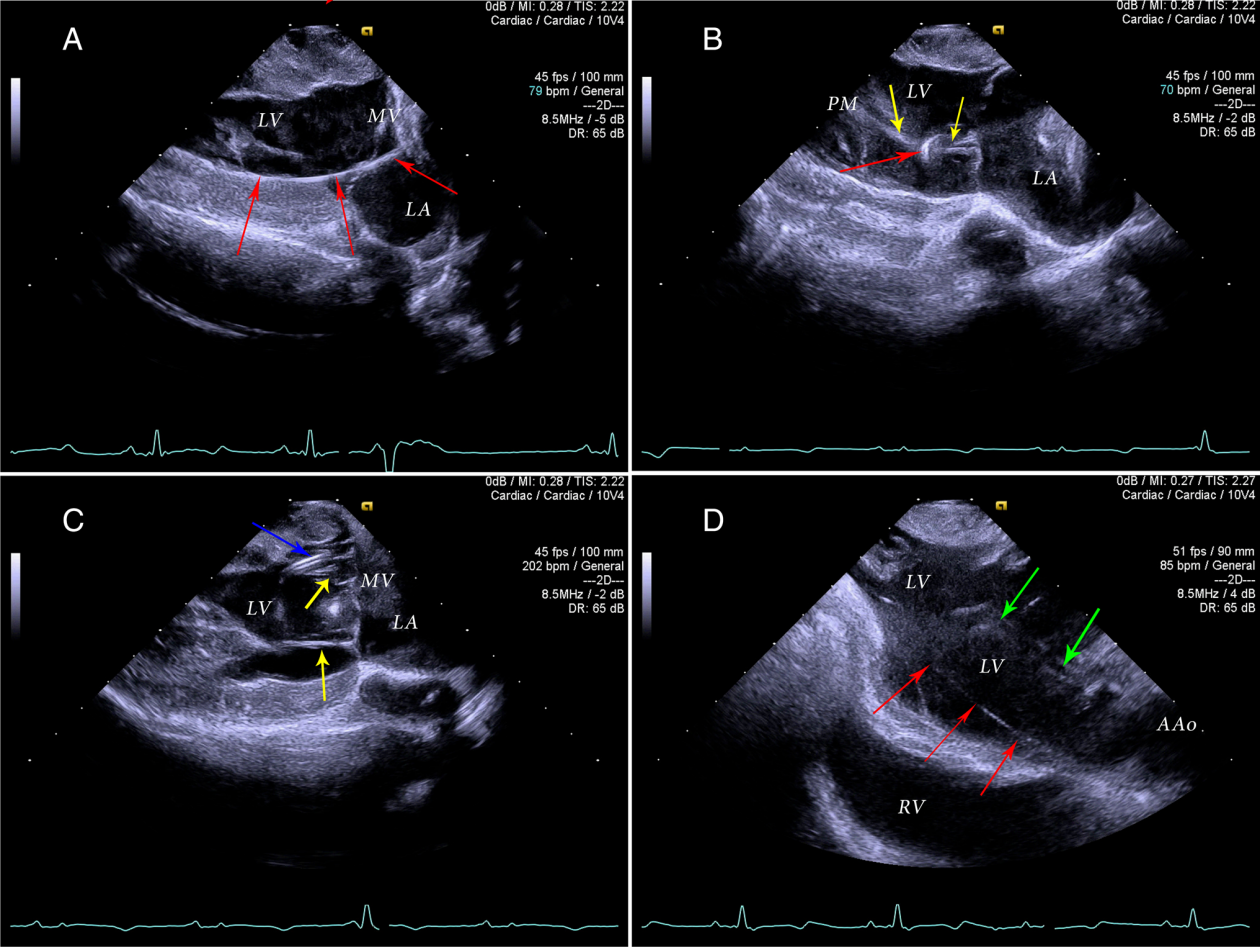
Echocardiographic two-dimensional epicardial imaging of malpositioned insetion kit elements of the Impella. LV – left ventricle, LA – left atrium, RV – right ventricle, MV – mitral valve, PM – papillary muscle. A Longitudinal view of the left ventricle and left atrium with stiff 0.035″ J-tip guidewire looping at the LV apex and propagating retrograde into the LA via MV (red arrows). Based on the findings, the wire was withdrawn until de-looped prior to the “railroad” insertion of the diagnostic catheter. B Longitudinal view of the LV and LA similar to the parasternal long-axis view obtain by transthoracic echocardiography. The diagnostic catheter has been introduced into position within LV cavity (yellow arrows) over the stiff 0.035″ guidewire, which was excessively pushed into LV during manipulations and looped around diagnostic catheter (red arrow). Partial withdrawal of the diagnostic catheter was required to allow for the J-wire to be carefully removed, avoiding intraventricular knotting. C Longitudinal view of the LV and LA following insertion of the thin soft 0.018″ wire via the diagnostic catheter (blue arrow). The diagnostic catheter is well-positioned, while the thin guidewire is excessively inserted, resulting in multiple loops within LV cavity and around mitral subvalvular apparatus (yellow arrows). Careful assessment of the thin guidewire placement is required due to the similar echocardiographic appearance of the device and of the mitral valve chordae, which may lead to the misidentification of parallel structures. D Modified longitudinal view of the apical and mid-portions of the LV following placement of the Impella over the soft 0.018″ guidewire. The position of the Impella is adequate (green arrows), while the guidewire is seen looping at the LV apex and propagating towards the ascending aorta (red arrows). Careful withdrawal of the guidewire is required to avoid kinking of the wire at the entry point of the Impella, which may prevent further wire evacuation and would warrant the removal of entire device
